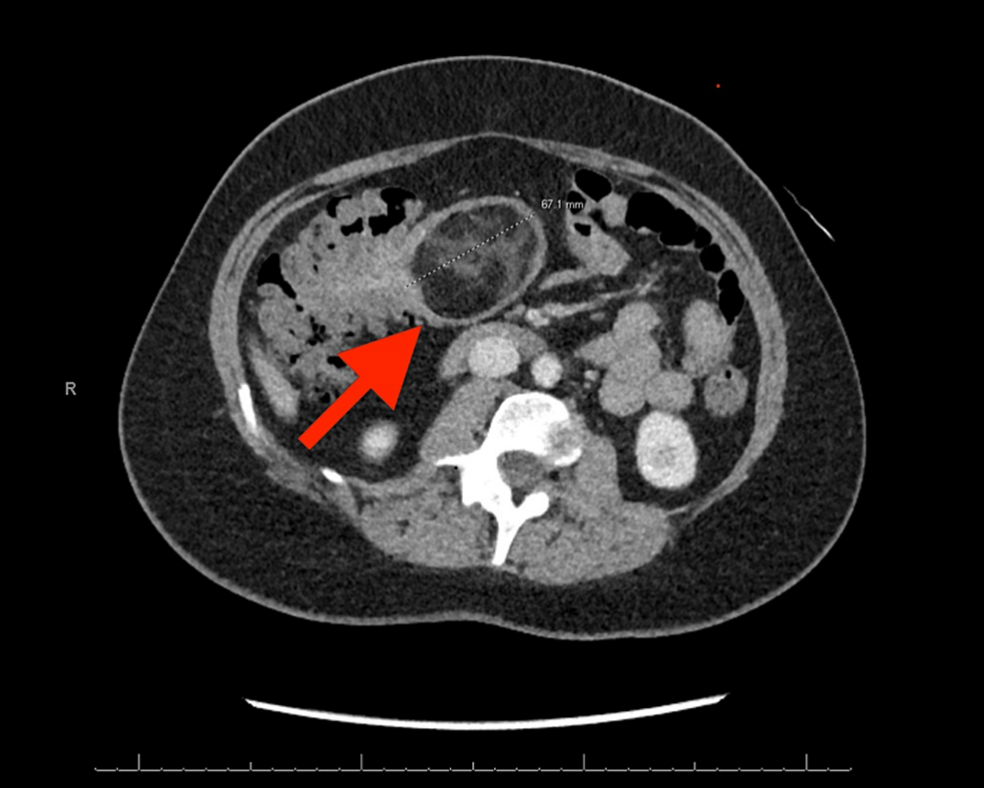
Contrast-enhancing CT axial view demonstrating lipoma as the lead point for ileocolic intussusception (red arrow) CT: computed tomography
